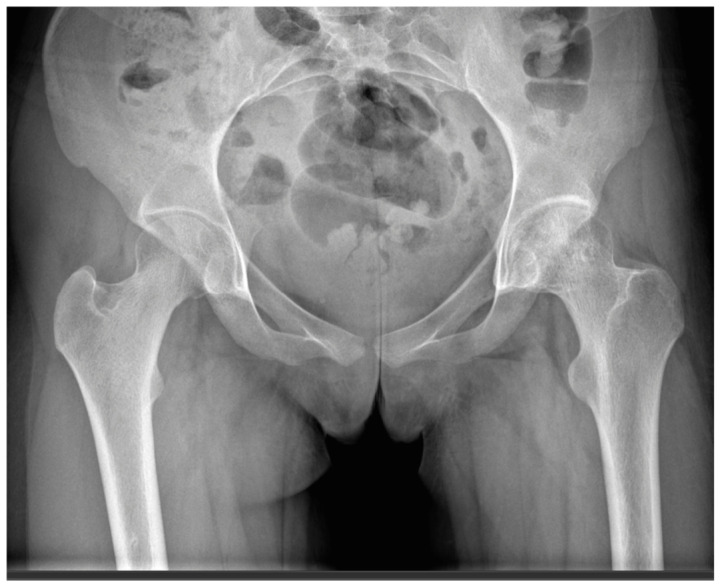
Postoperative AP pelvic radiograph taken at 3 years of age. The image shows preserved articular surface congruence. There are no signs of AVN.
